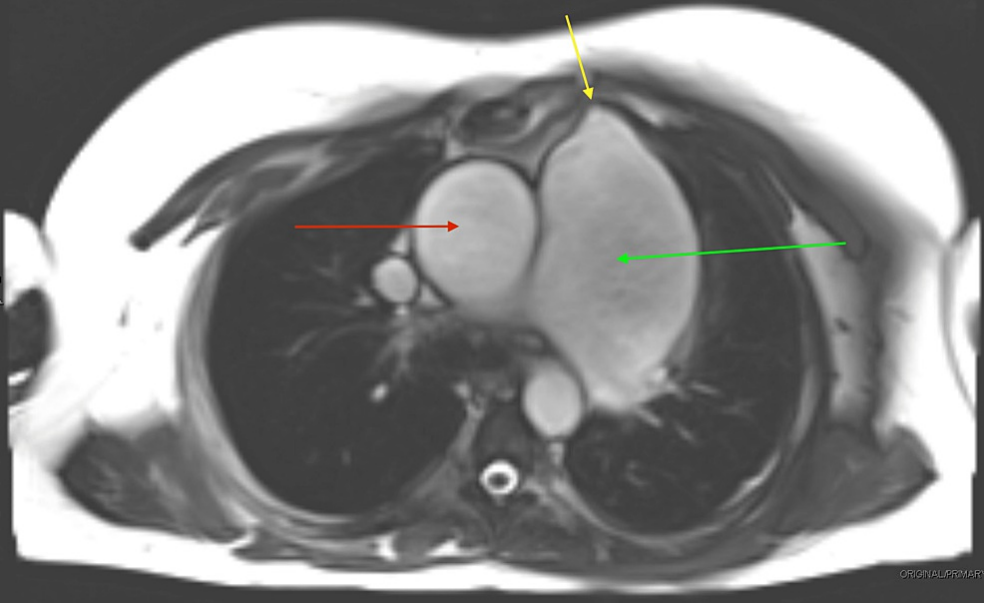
Morphological cardiac MRI (1.5 T with gadolinium contrast) showing erosion of the PAA into the sternum Yellow arrow: pulmonary artery eroding into the sternum Green arrow: PAA as previously appreciated Red arrow: aortic aneurysm
